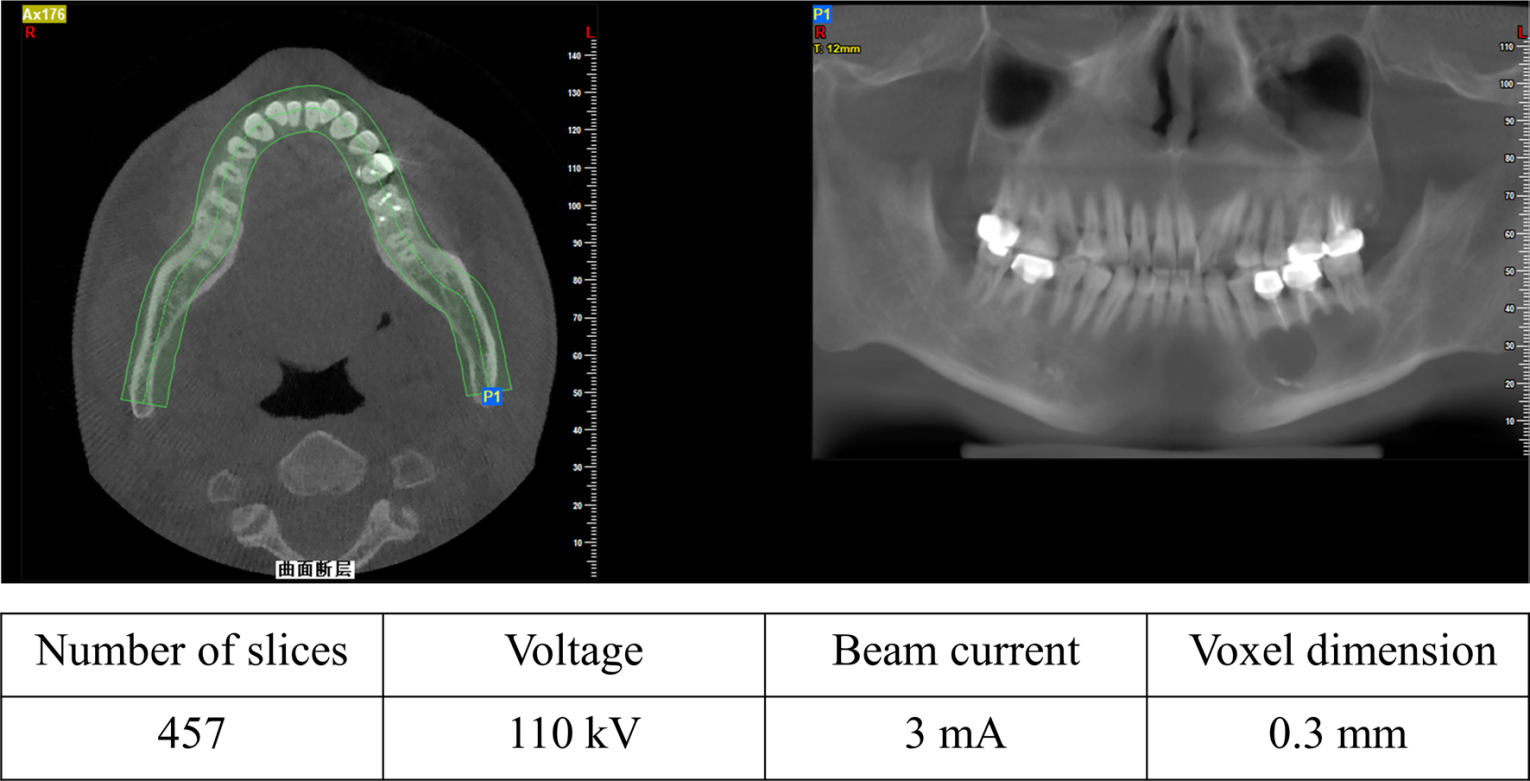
Typical CBCT images of periapical cysts after restoration
